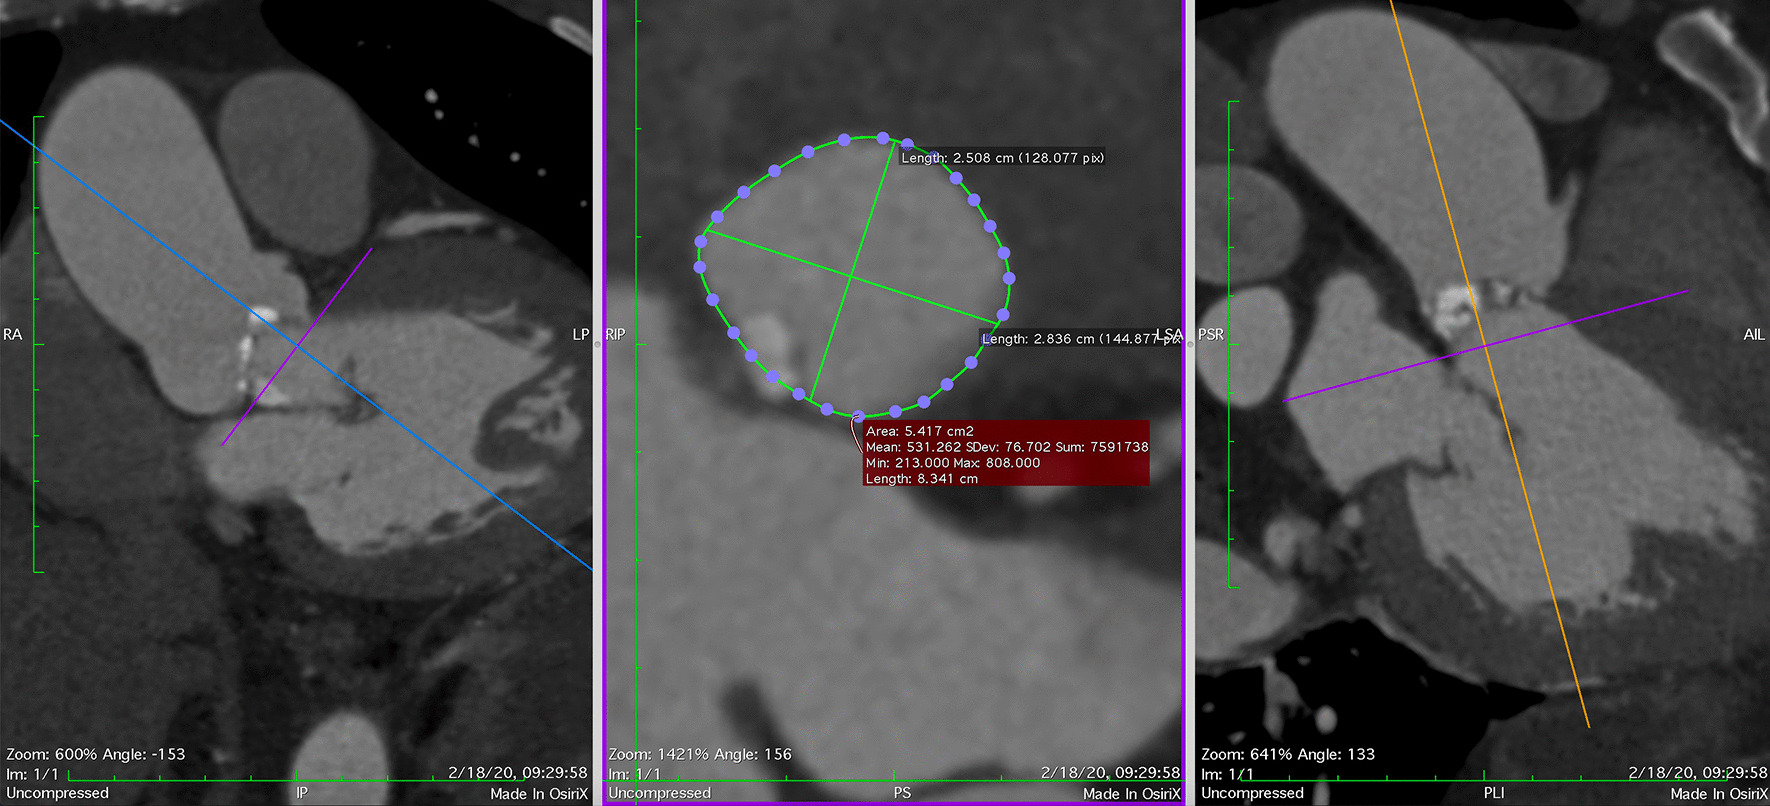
Measurement of aortic annulus diameters on CT scan
